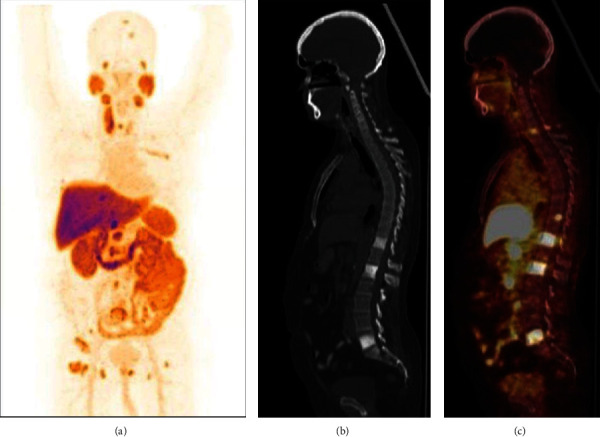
^18^F-PSMA-1007 (PET-CT) scans: (a) maximum intensity projection (MIP) PET image, (b) noncontrast CT (sagittal) image, and (c) PET-CT fused sagittal image showing multiple PSMA-avid lesions in bone (skull, spine, and right femur) and right-side cervical nodes.
